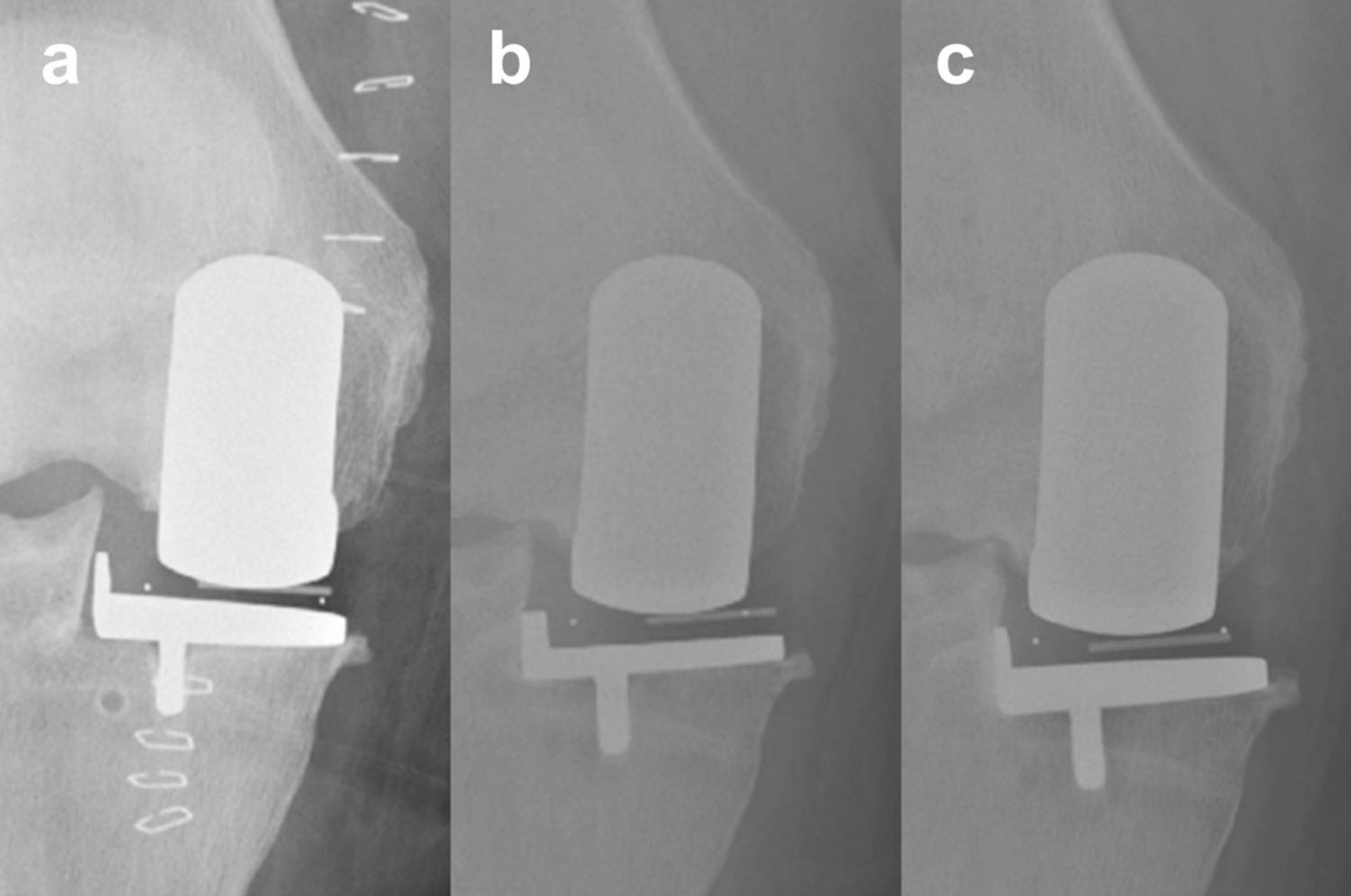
Representative radiographs of tibial component subsidence. (a) Immediate postoperative radiograph. (b) Radiograph at the first outpatient follow-up, showing valgus subsidence of the tibial component. (c) Radiograph at 1 year postoperatively, demonstrating no further progression of subsidence
